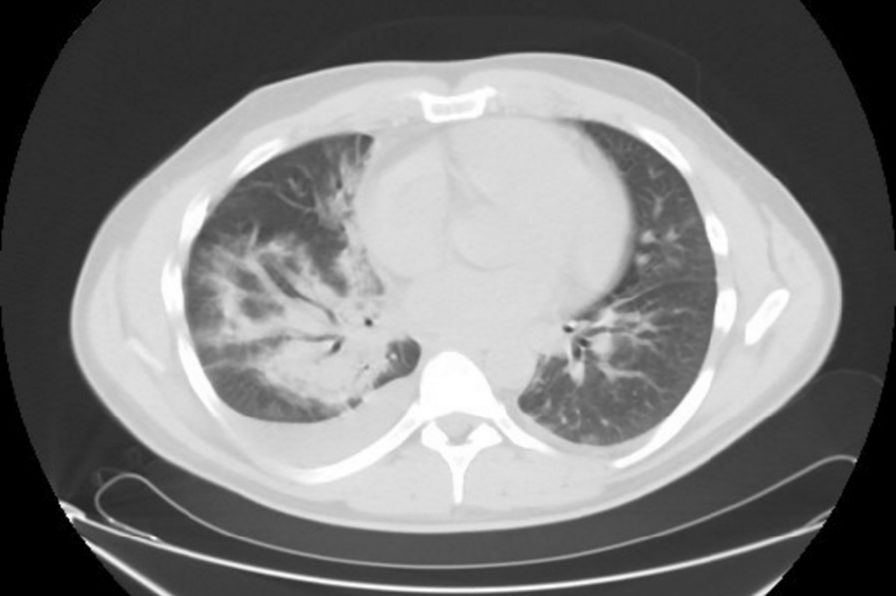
Chest computed tomography during malaria *Plasmodium ovale* disease. Chest computed tomography showed interstitial bilateral pneumonia with consensual pleural effusion
